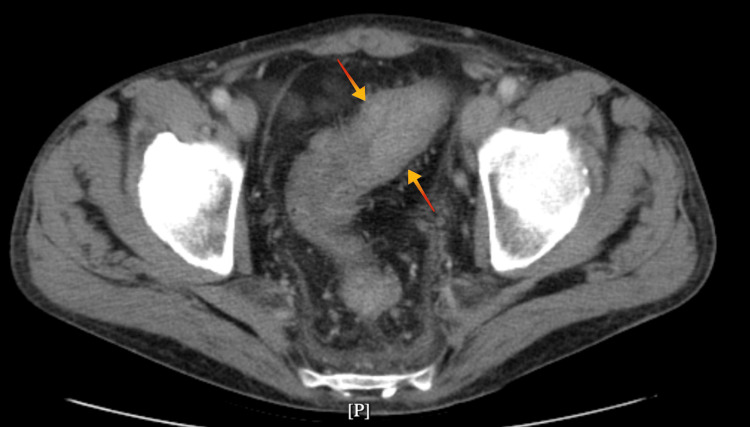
CT scan of the abdomen showing edematous and thickened bowel wall with contrast enhancement (arrows).
